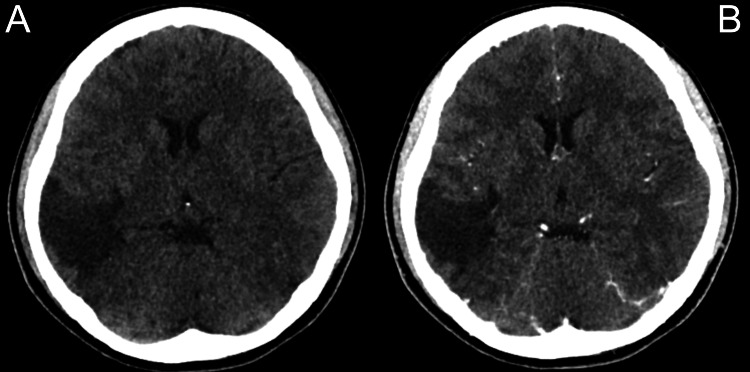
CT scan of the brain, plain (A), and contrasted (B) showing right parieto-temporal lobe encephalomalacia.
